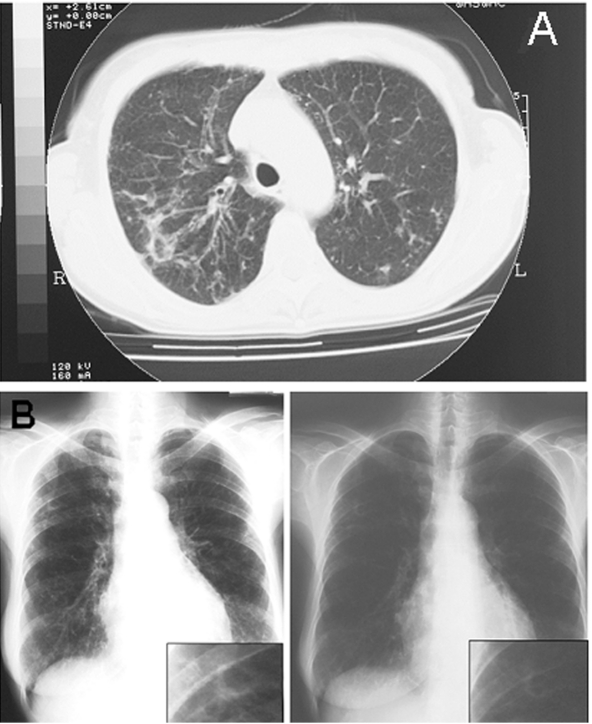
A) Pulmonary computed tomographic scan representation of *Mycobacterium lentiflavum* lesions. Radiologic image shows the appearance of a widespread reticulonodular alteration and an opacity in the left middle lobe. B) Chest radiograph evolution after 3 months of treatment shows a sustained improvement of the radiologic alterations to the left pulmonary middle lobe.
